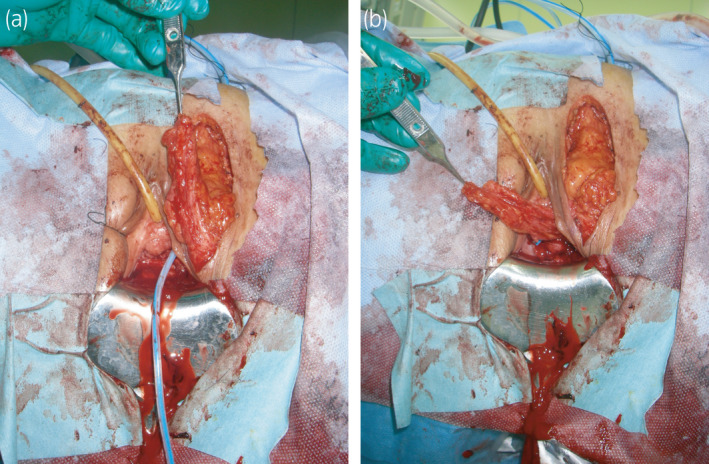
Second UVF closure with a Martius flap. (a) A skin incision was made in the left labium. The Martius flap was made while maintaining an adequate blood supply (monitored with Doppler ultrasound). (b) A tunnel was created between the left labium and the vaginal incision. The Martius flap was inserted into the tunnel and interposed between the urethra and the vaginal wall.
